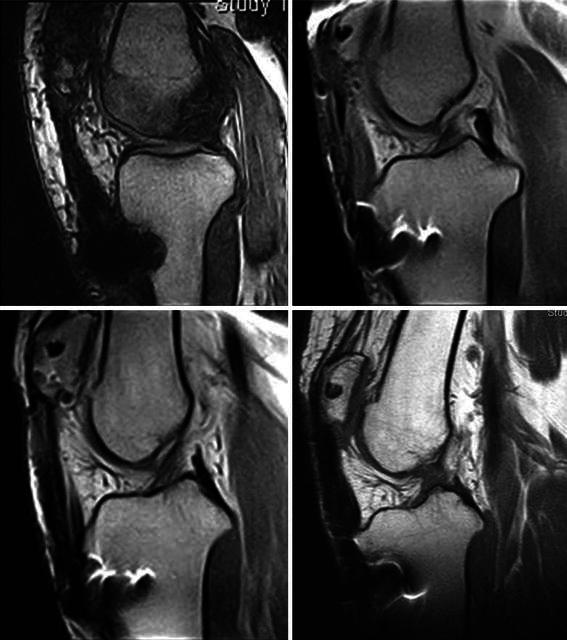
MRI images at 3, 6, 12, and 24 months postop, showing the evolution of the signal of the reconstructed patellar tendon
